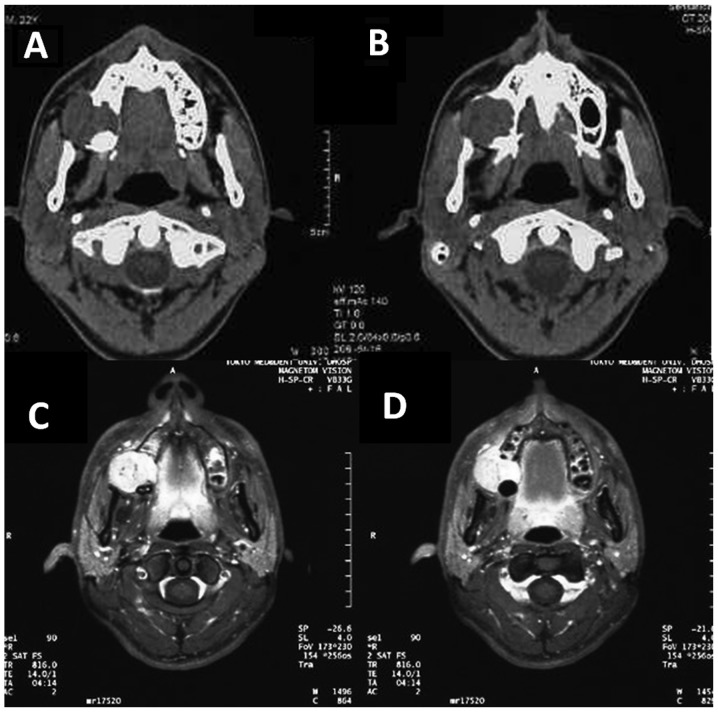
(A and B) Computed tomography (CT) and (C and D) Axial contrast-enhanced magnetic resonance imaging (CE-MRI). (A) Axial CT image revealing a globular-shaped lesion arising from the inside maxillary bone with destruction of its posterior wall and alveolar bone. (B) The lesion exhibited intermediate signal intensity on T1-weighted imaging, and heterogeneous high signal intensity on T2-weighted and short TI inversion-recovery imaging. (C and D) CE-MRI revealed a distinctly-bordered lesion (31×30 mm) extending from the right maxillary alveolar process to the right palate and reaching the retromaxillary fat space.
